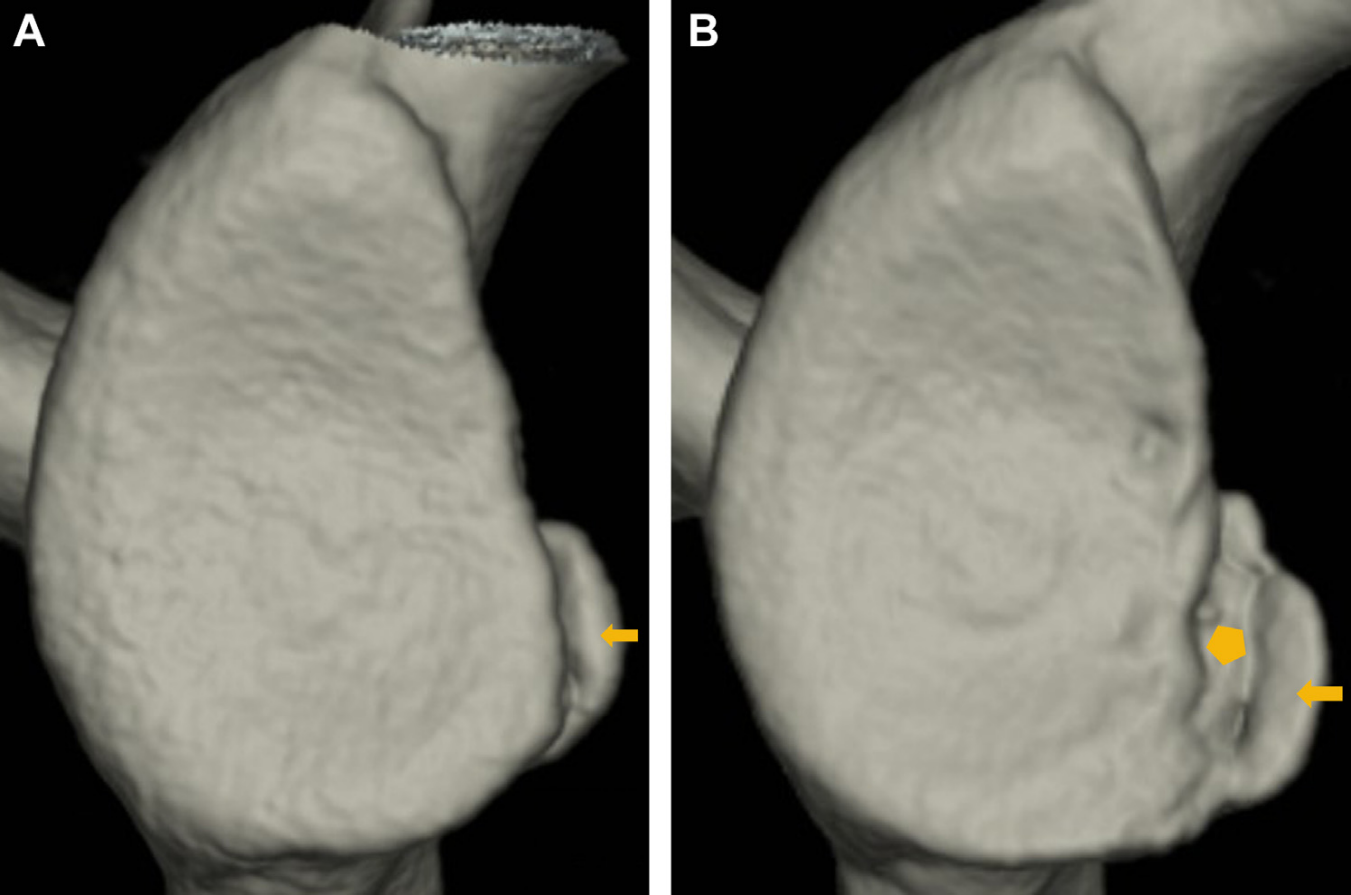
An American football player with a new glenoid fracture at second CT (right shoulder). (A): First CT at 6 months after primary instability (16 years old, once instability event). (B): Second CT at 4 years after primary instability (20 years old, total 4 instability events). At the first CT, he came to our hospital because of instability in left shoulder and did not complain an apprehension in right shoulder. At the second CT, he complained of an apprehensive sensation in right shoulder. A new glenoid fracture () accompanied with a previous bone fragment () was recognized. *CT*, computed tomography.
